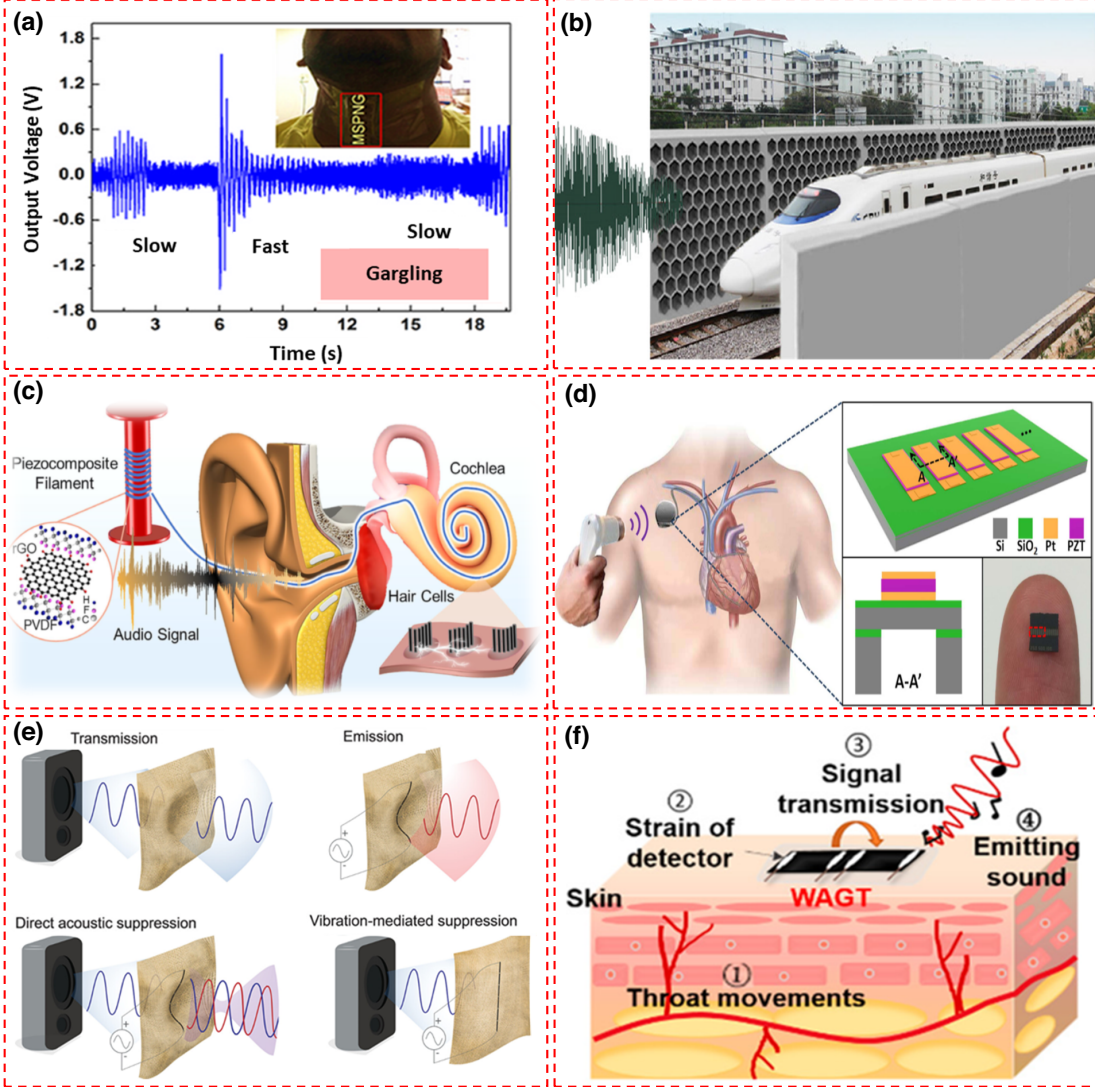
Applications of Acoustic Piezoelectric Nanogenerators. a Throat-attached nanogenerator used for self-powered wearable devices, capable of detecting vibrations from vocal activities like speaking or gargling, enabling real-time monitoring^57^. Copyrights © 2019 Elsevier Ltd, all rights reserved. b Noise reduction and energy harvesting in high-speed railways^81^. Copyright 2018 Elsevier Ltd. All rights reserved. c Nanostructured piezoelectric filaments for next-generation cochlear implants^88^. Copyright © 2016, The Author(s), all rights reserved. d Ultrasonic activation of PTFE particles for sonodynamic cancer therapy^87^. Copyright © 2024 Wiley & Sons, all rights reserved. e Multifunctional fabrics engineered for both sound emission and suppression, offering innovative solutions for noise control and active sound management^89^. Copyright © 2024 Wiley-VCH GmbH, all rights reserved. f Wearable artificial graphene throat for assistive communication, designed to detect throat movements and convert them into sound signals for improved communication capabilities^90^. Copyright © 2019 American Chemical Society, all rights reserved
